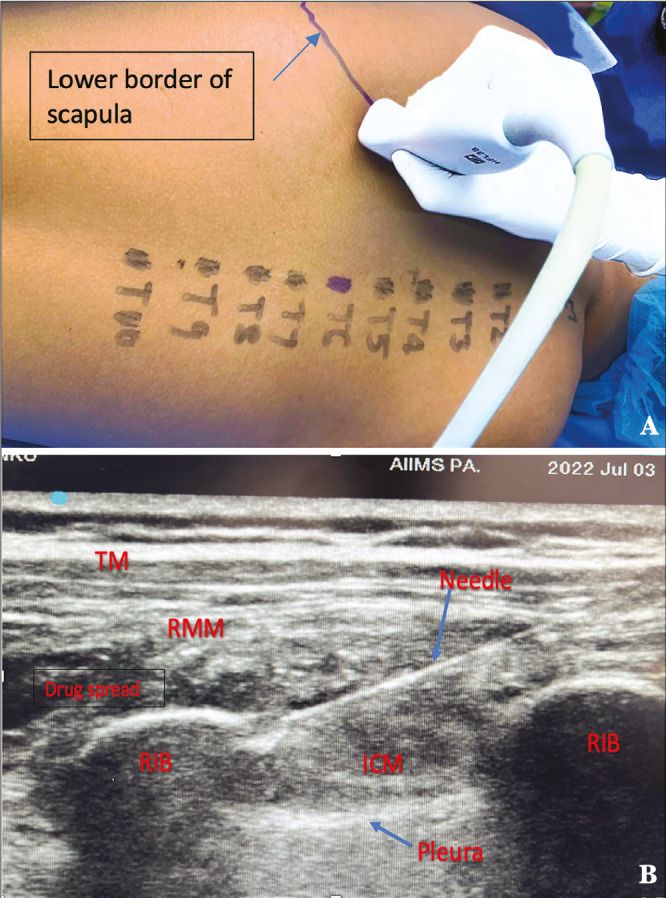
Rhomboid intercostal block. A: Placement of ultrasound probe for administration of rhomboid intercostal block; B: The Landmarks of rhomboid intercostal block and local anaesthetic injection under ultrasound view. TM, Trapezius muscle; RMM, Rhomboid major muscle; ICM, Intercostal muscle; RIB, rhomboid intercostal blocks.
